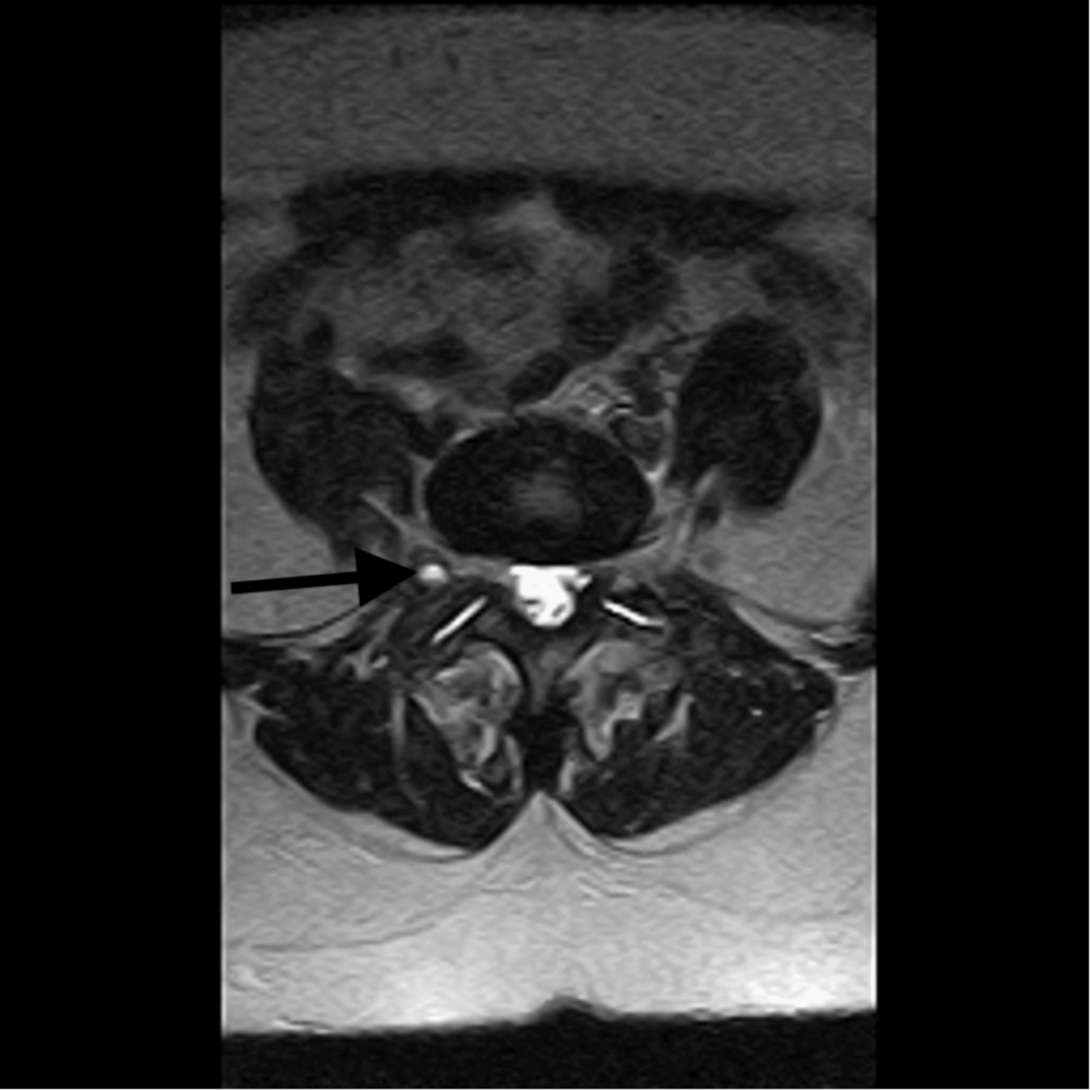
Axial MRI view of the lumbar spine for Case 1. Black arrow indicates a synovial cyst at L5-S1 with adjacent fluid causing instability. L5: Lumbar Segment 5; S1: Sacral Segment 1.
